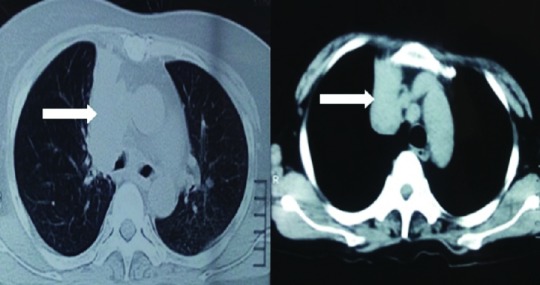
Chest computed tomography revealing presence of right para-hilar lung mass (white arrows).
